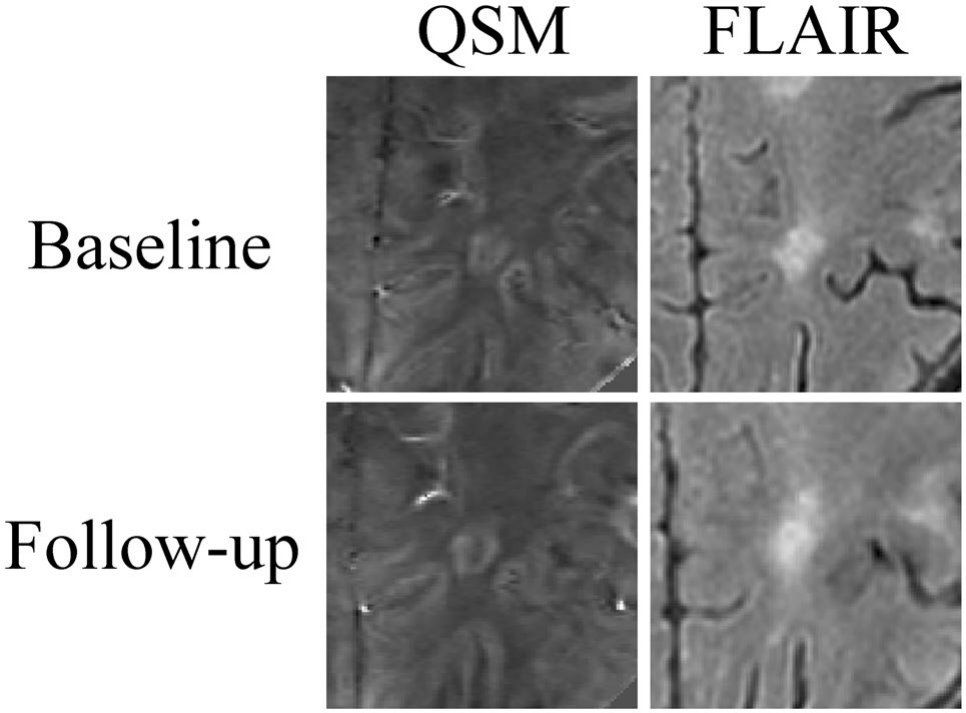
Representative baseline and follow-up fluid-attenuated inversion recovery (FLAIR) and quantitative susceptibility mapping (QSM) images showing a persisting paramagnetic rim lesion. QSM images are windowed from − 0.1 to 0.2 ppb
